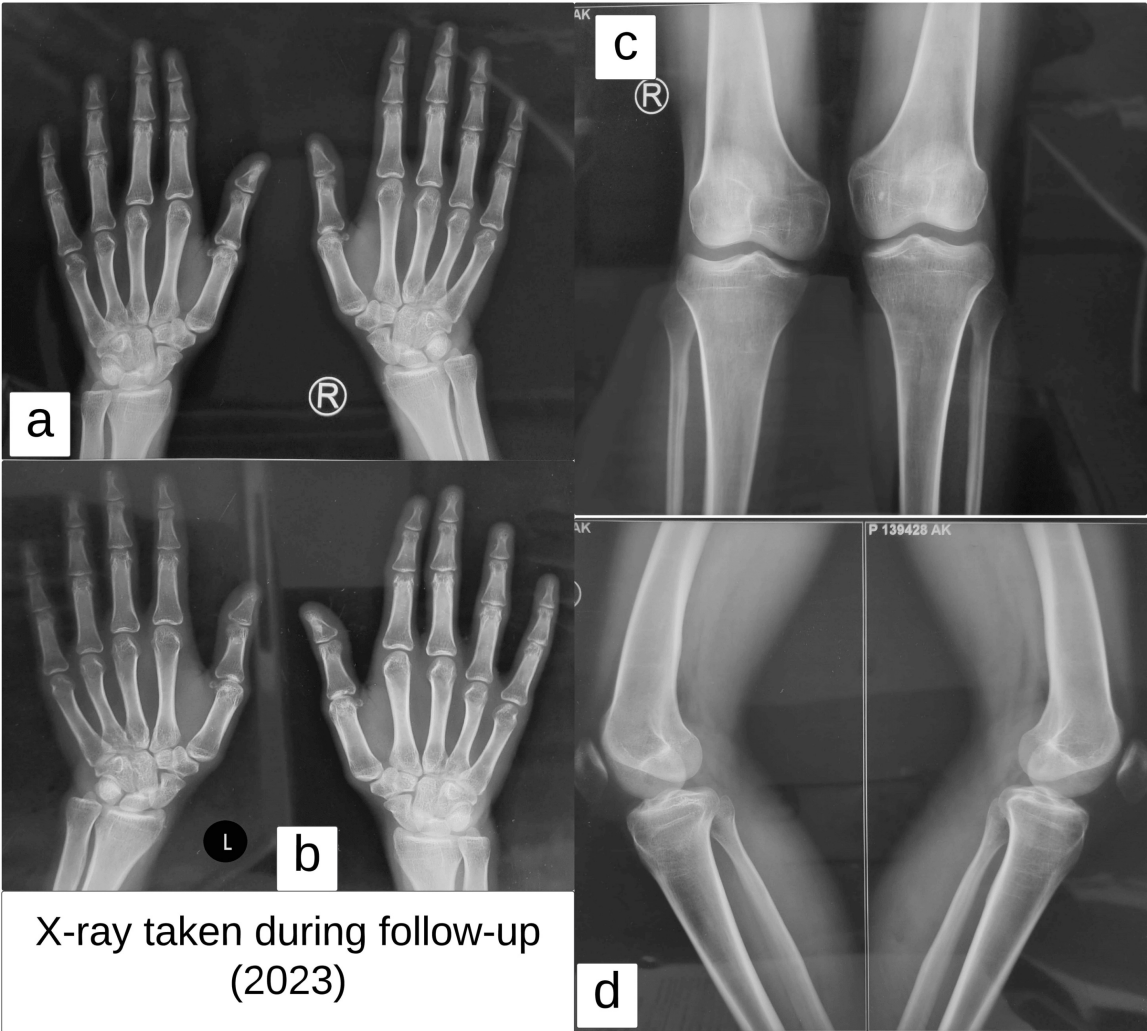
Follow up X-ray showing (a) and (b) X-ray hand and wrist; (c) and (d) X-ray bilateral lower limb showing marked improvement in the previously widened, frayed, and cupped metaphysis of long bones, with the meta-diaphyseal region appearing significantly more uniform, genu valgum showing partial correction (c) and (d), and there is also a significant increase in bone density compared to the previous X-ray (Figure 4)
